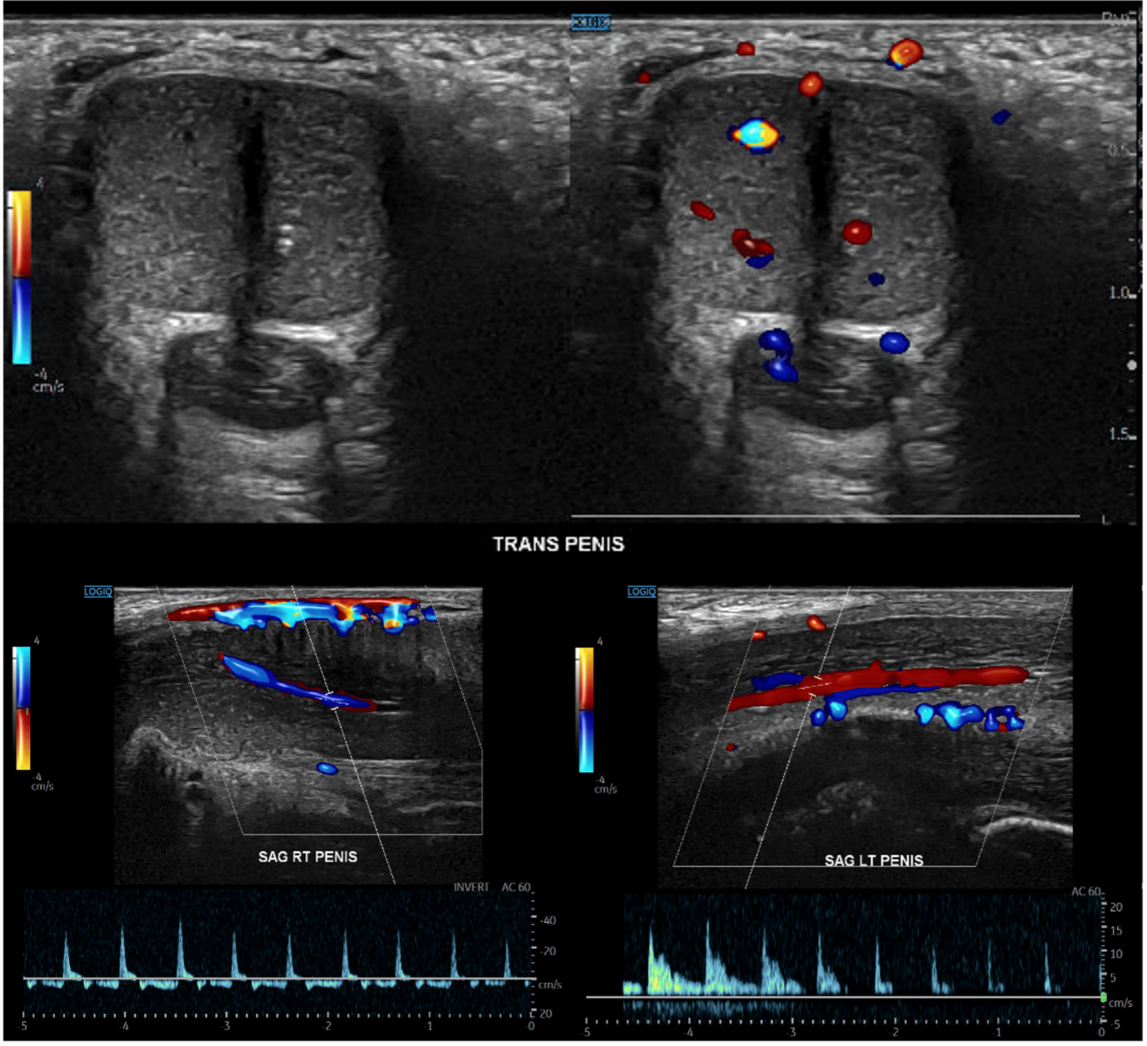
Doppler evaluation Penile Doppler ultrasonography demonstrated preserved arterial and venous blood flow without evidence of thrombotic complications or vascular compromise.
